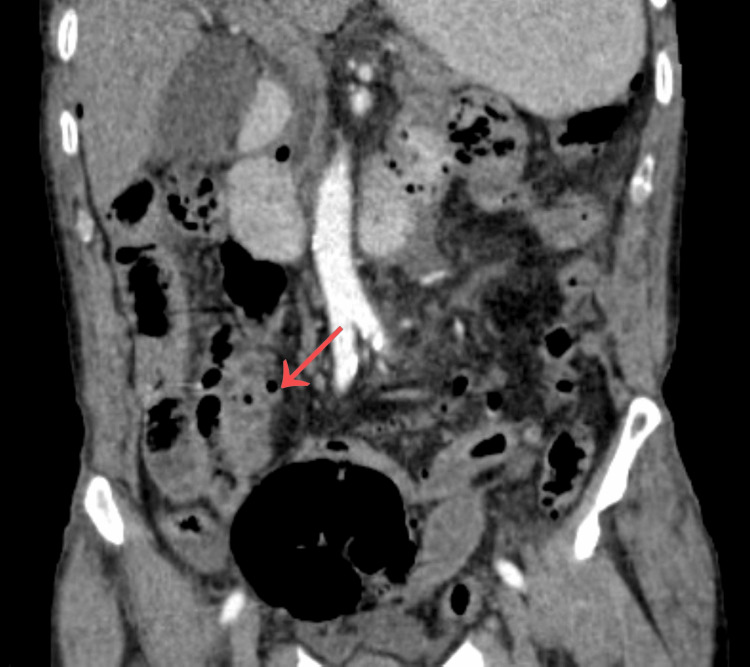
Ileal diverticula with inflammatory changes on computed tomography. Coronal contrast-enhanced computed tomography image demonstrating ileal diverticular disease. The ileal diverticular outpouching is indicated by the arrow, with adjacent segmental bowel wall thickening and surrounding inflammatory fat stranding corresponding to the inflammatory changes described in the text.
